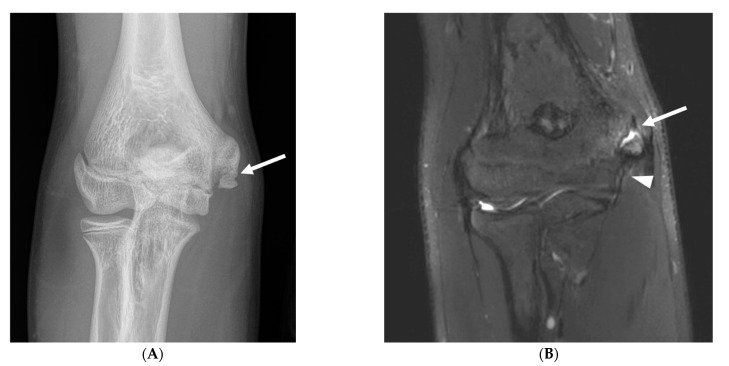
A 12-year-old right-hand-dominant male baseball pitcher who developed acute medial elbow pain during a pitching lesson. (A) AP radiograph demonstrates an acute avulsion fracture of the medial epicondylar apophysis (arrow). (B) Coronal fat-saturated T2-weighted image demonstrates an acute avulsion fracture of the medial epicondylar apophysis (arrow). The anterior band of the ulnar collateral ligament remains intact (arrowhead).
